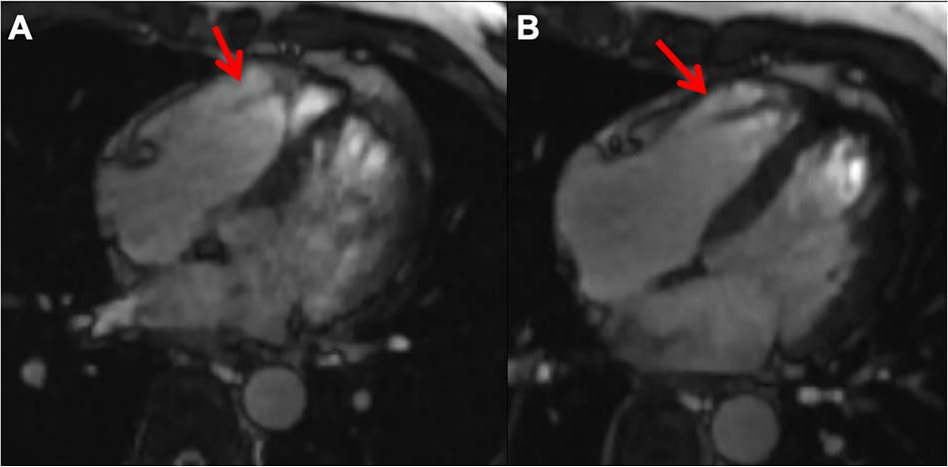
Cine cardiac MRI snapshots capturing the suspected vegetation site. In Panel (A), the image reveals the papillary muscle crossing the moderator band. Panel (B) highlights the extension of the papillary muscle towards the tricuspid valve leaflet, confirming it as normal structure. Arrowheads denoting the papillary muscle.
